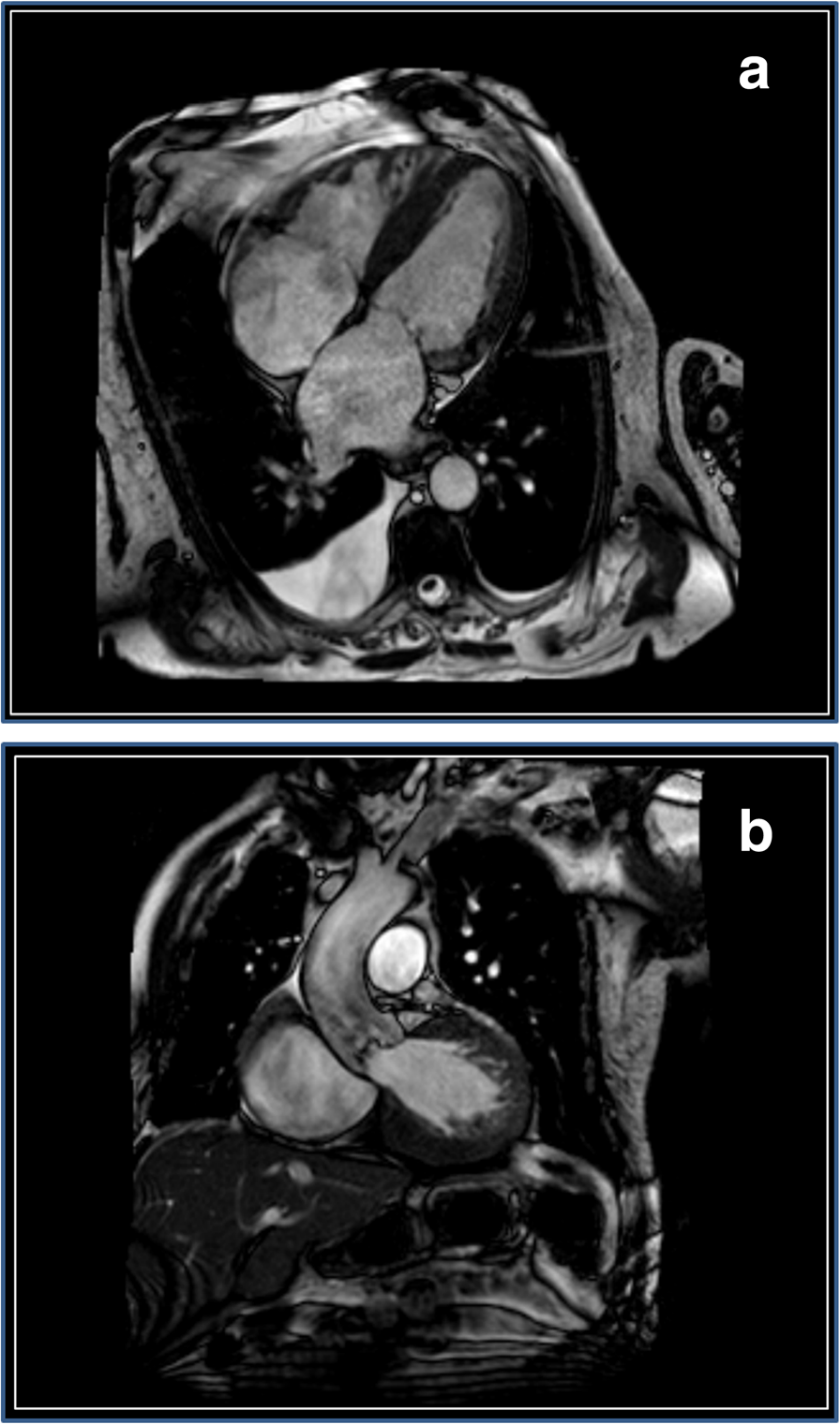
a Native MRI, 4-chamber view, showing enlarged atria und left ventricular hypertrophy (LA 40 mm^2^; RA 41 mm^2^, lateral wall thickness 14 mm; septal wall thickness 16 mm) as signs of cardiac amyloidosis. b Morphologic aspect of the left ventricular outflow tract and aortic valve in MRI
